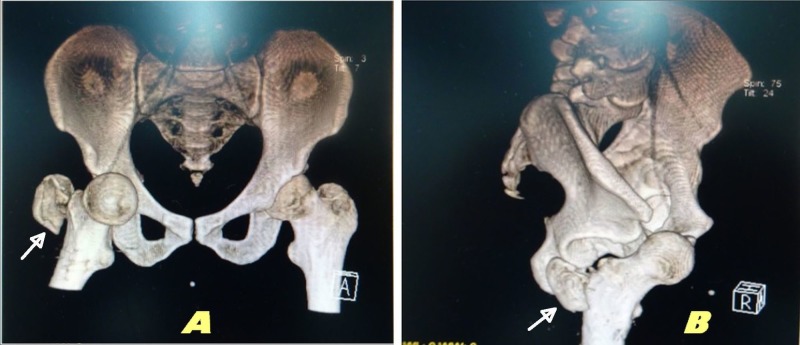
Computed tomography (CT) scan of the pelvis with three-dimensional (3D) reconstruction from the anterior aspect (A) and right lateral aspect (B) Pubic-type anterior dislocation of the right hip with ipsilateral displaced greater trochanter fracture (white arrows).
